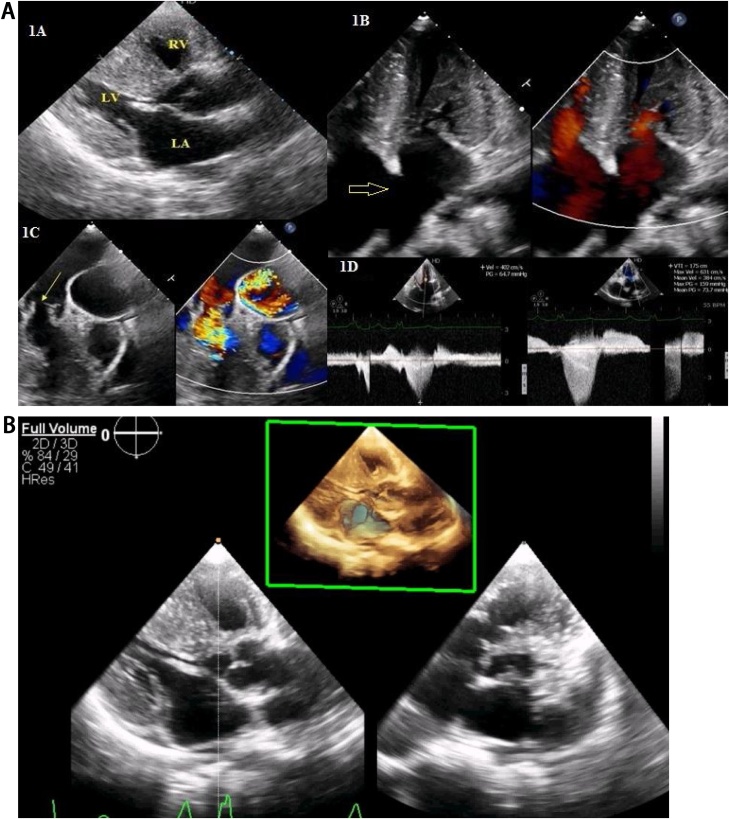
A: 2D-Echocardiography parasternal long axis showing biventricular hypertrophy. B: 2D-Echocardiography apical four-chamber showing biventricular hypertrophy, Large ASD (arrow), and color Doppler showing left to right shunt. C: 2D-Echocardiography parasternal short axis showing, dysplastic pulmonary valve (arrow), and severe pulmonary stenosis. D: 2D-Echocardiography showing LV gradient of 64.7 mm and peak pulmonary gradient of 149 mm. E: 2D and 3D-Echocardiography left parasternal long axis showing biventricular hypertrophy.
